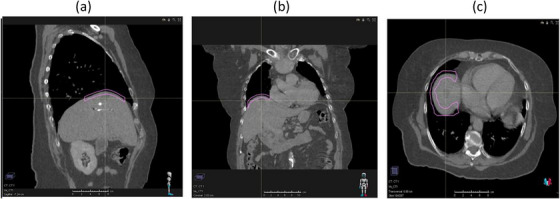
Diaphragm (pink) contoured on (a) sagittal, (b) coronal, and (c) axial CT view in TPS.
